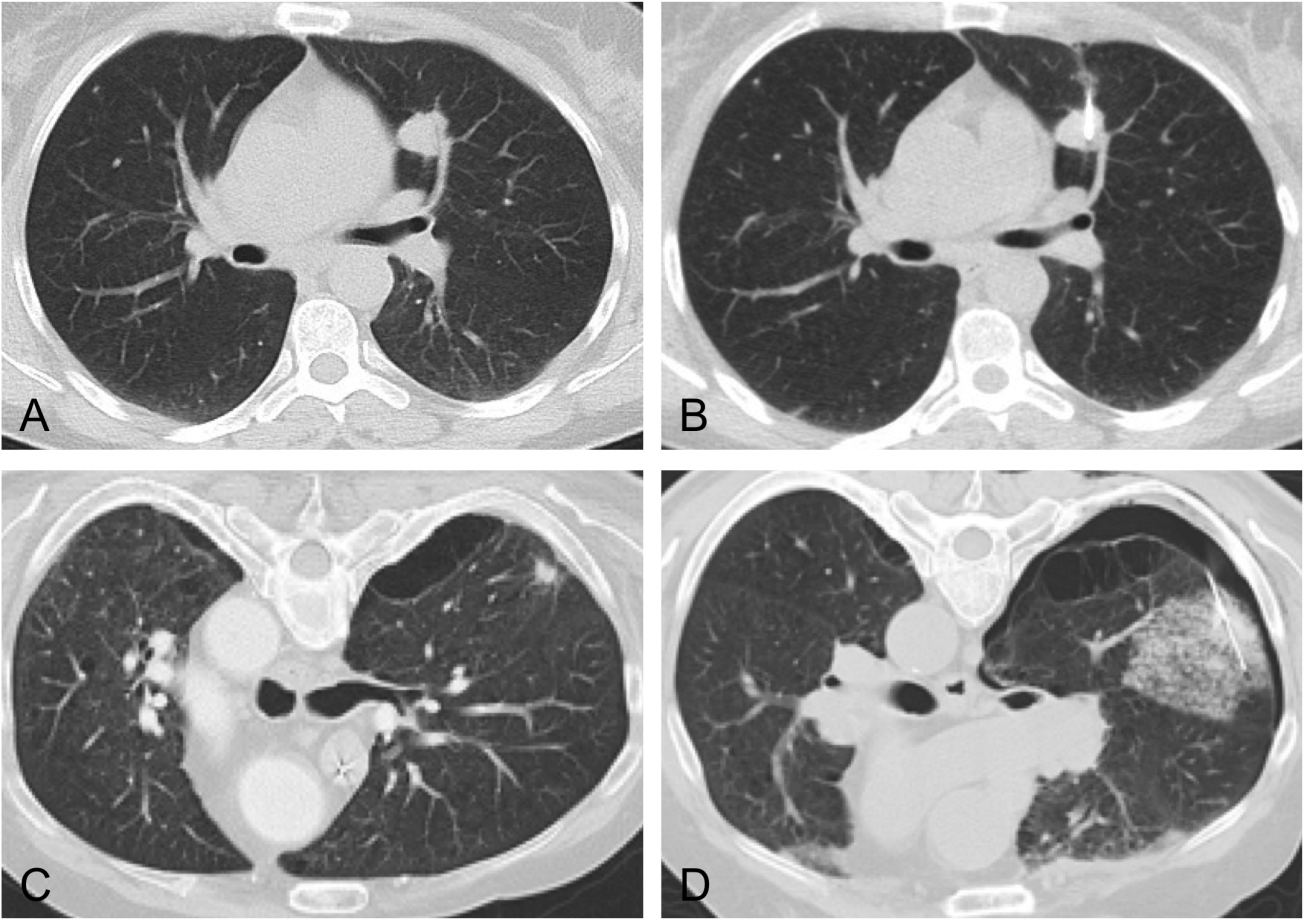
Exemplary images of CT-guided percutaneous transthoracic pulmonary biopsies with and without lung emphysema. Pulmonary nodule in otherwise normal lung parenchyma before (A) and after PTPB (B). No post-interventional complication was detected. Pictures (C) and (D) show a patient with pulmonary emphysema. After PTBP (D) CT examination revealed pneumothorax and parenchyma hematoma.
